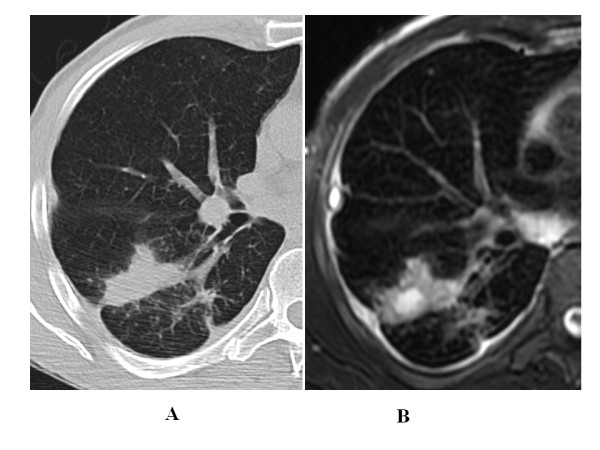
38 year-old man with pulmonary tuberculosis. A) HRCT shows parenchymal consolidation. B) Unenhanced MRI depicts colliquative necrosis within consolidation, subtle, free and loculated pleural effusion, and a highly hyper-intense mediastinal lymph node.
